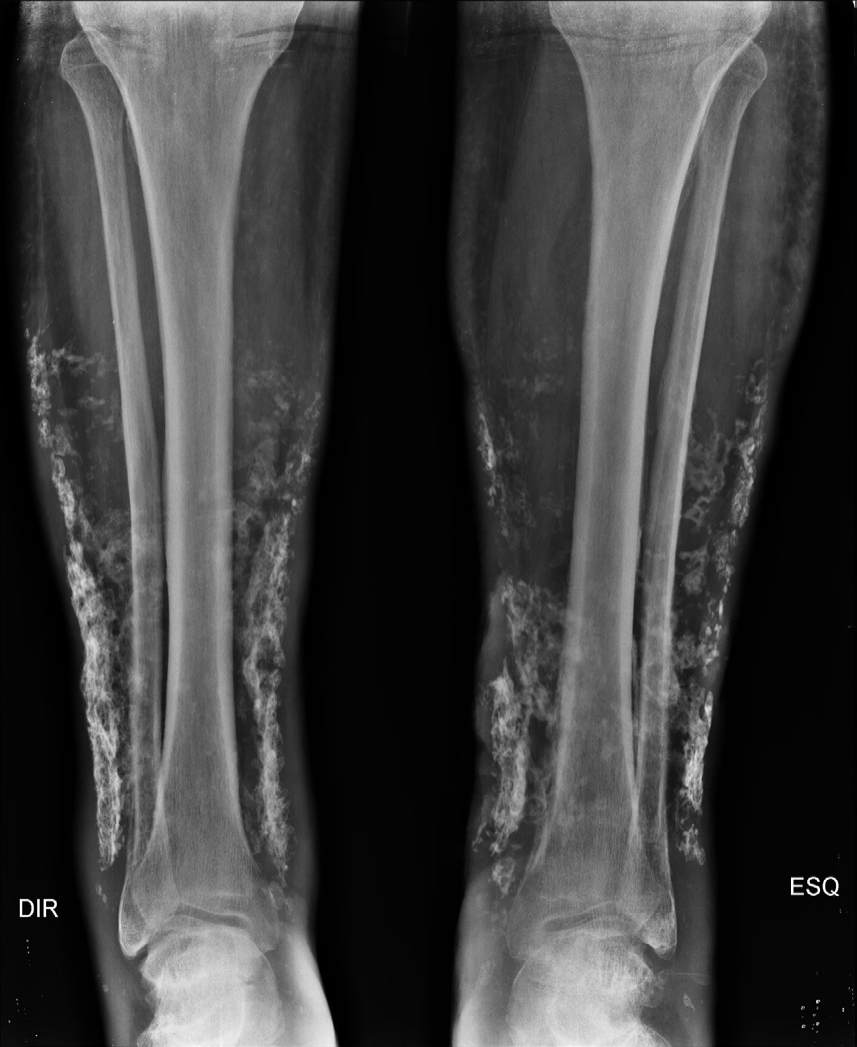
Simple X-rays of the lower limbs: lesions compatible with amorphous calcifications of soft tissues of the external aspect of the mid and lower third of the legs.
